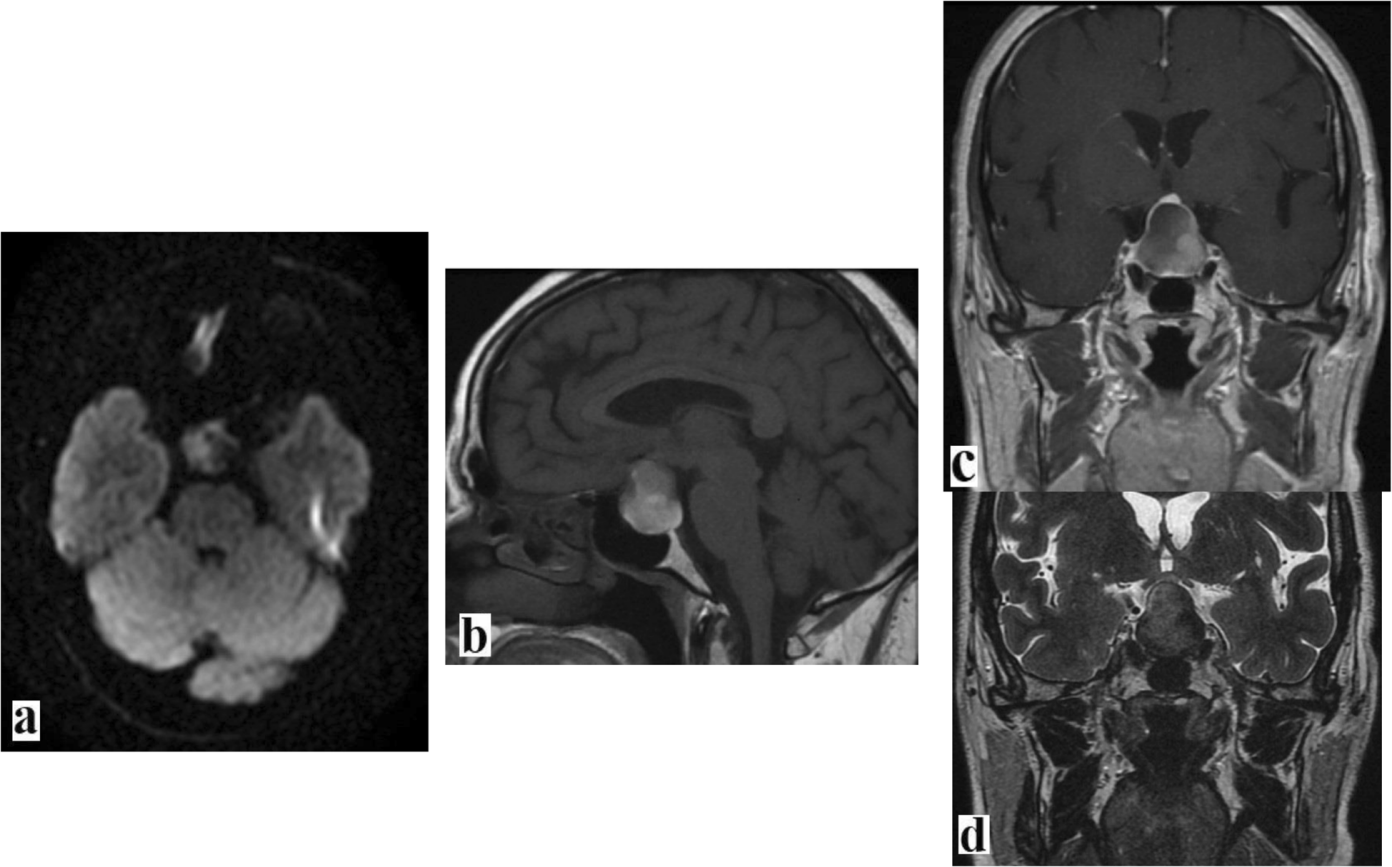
Brain magnetic resonance imaging scans. a Diffusion-weighted imaging (DWI). b T1w-weighted (T1w) imaging. c T1w + gadolinium. d T2-weighted (T2w) imaging. Images show an enormous pituitary mass exhibiting atypical features such as a heterogeneous mixed high- and low-signal T1w and T2w with rim enhancement. DWI showed a mild and partial high intensity in the central part of the mass. These features suggest a mixed cystic and hemorrhagic nature of this pituitary abscess
